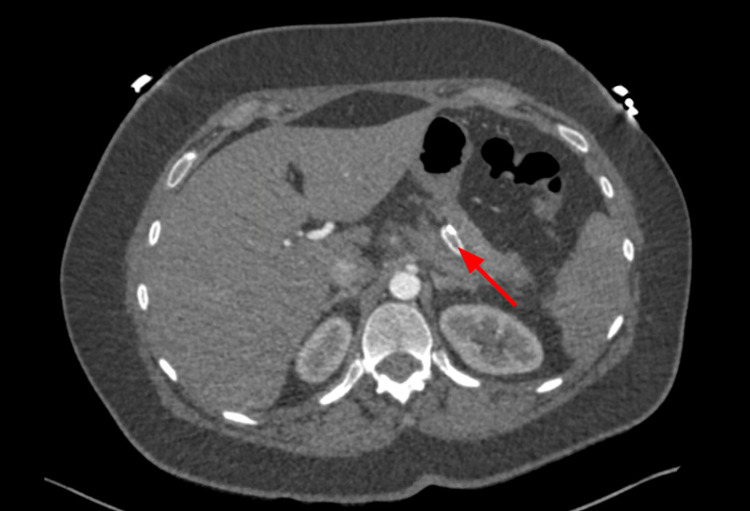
CTA abdomen and pelvis on POD#1 redemonstrating the thrombus in the splenic artery (red arrow). CTA, computed tomography angiography; POD, post-operative day
